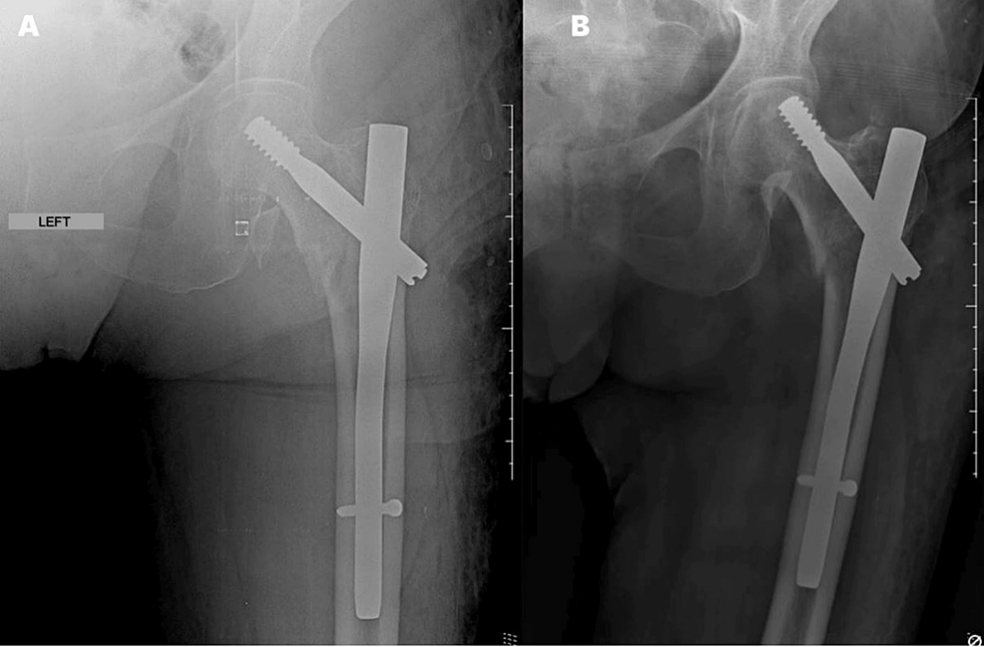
(A) Immediate postoperative radiograph and (B) after five weeks, showing cut-out.
